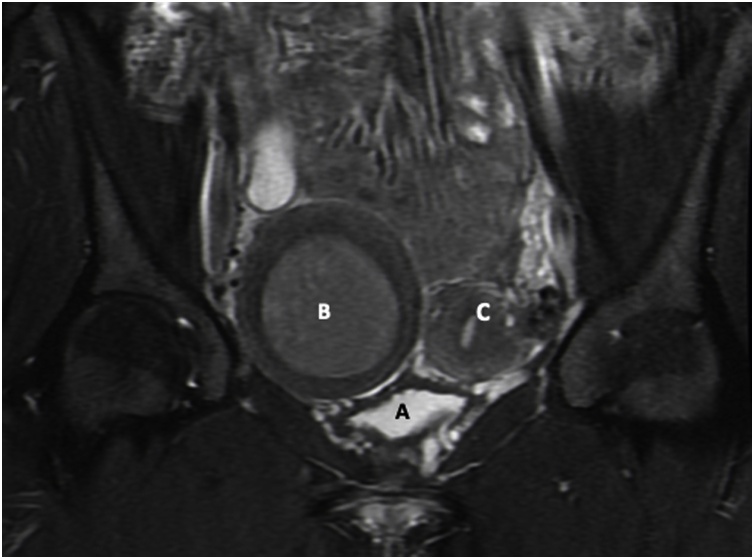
The coronary plane of MRI with IV contrast: A. Bladder, B. Hematometra in the rudimentary non-communicating horn with functioning cavity, misread as right ovarian cyst structure, C. Left unicornuate uterus.
